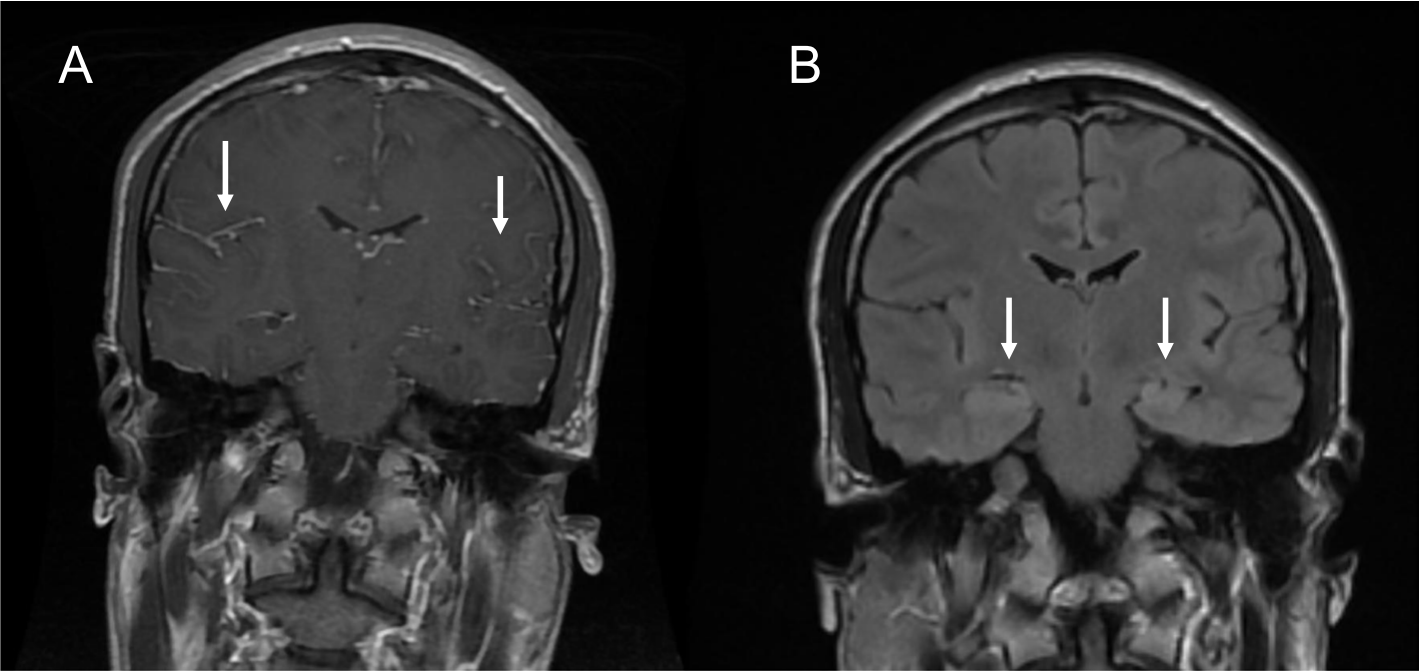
A Coronal brain MRI T1-weighted images with gadolinium contrast on April 13 (Day 0) demonstrate leptomeningeal enhancement (arrows). These findings were transient and were not demonstrated on an MRI scan on April 19 (Day 7). Usually, leptomeningeal enhancement is suggestive of infection, vasculitis or neurosarcoidosis; however, the results of a comprehensive screening for these conditions were normal. It was particularly important to exclude herpes CNS infection (HSV 1, HSV 2, HHV 6,7) because these infections can precede or coexist with AE [4]. B Coronal brain MRI T2-weighted FLAIR images on April 19 (Day 7) demonstrate bilateral hyperintensities in the medial temporal region (arrows), suggesting limbic encephalitis
